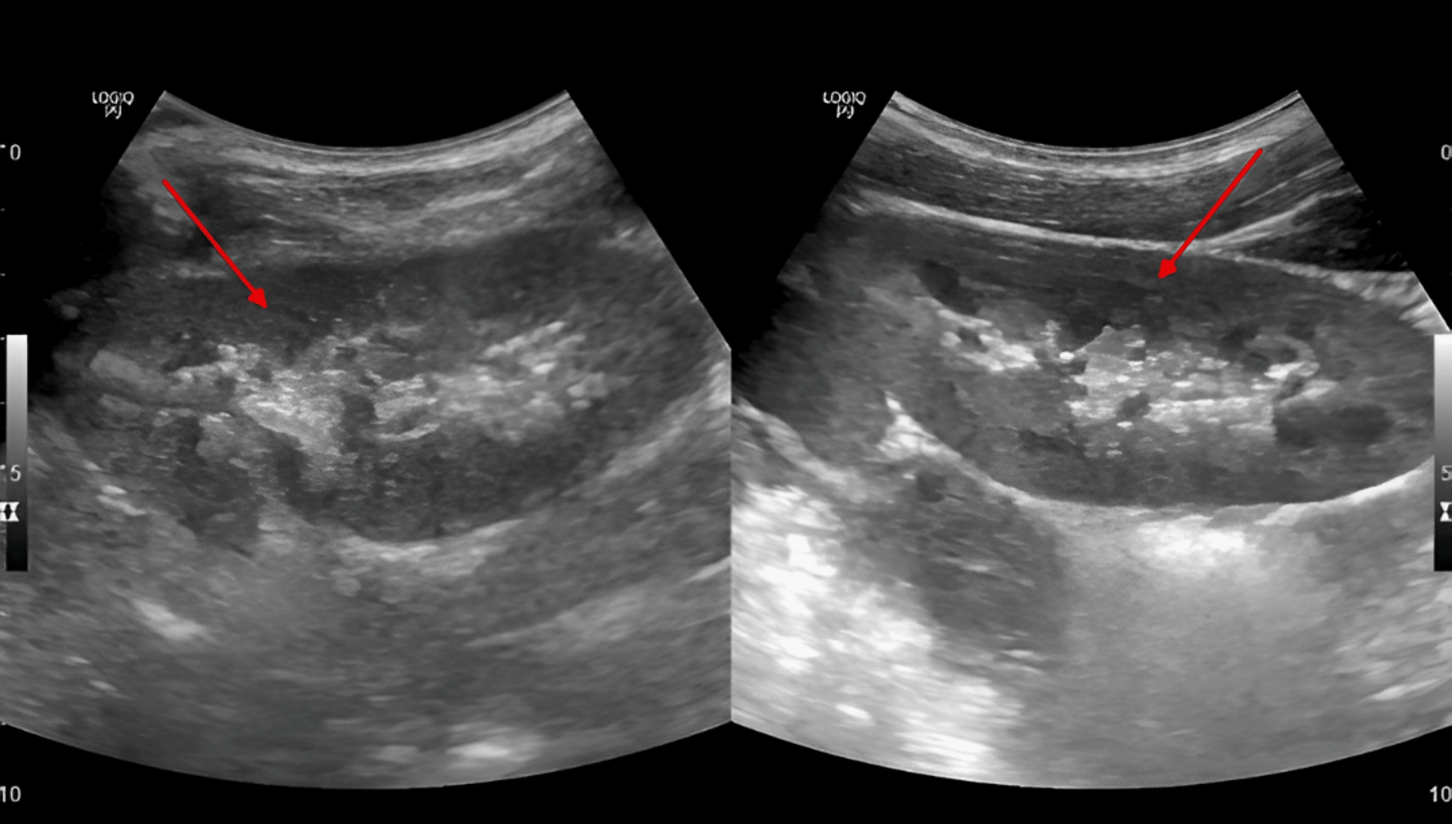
Renal ultrasound Revealed increased parenchymal echogenicity and partial loss of corticomedullary differentiation in both kidneys, findings suggestive of chronic renal inflammation or granulomatous disease
